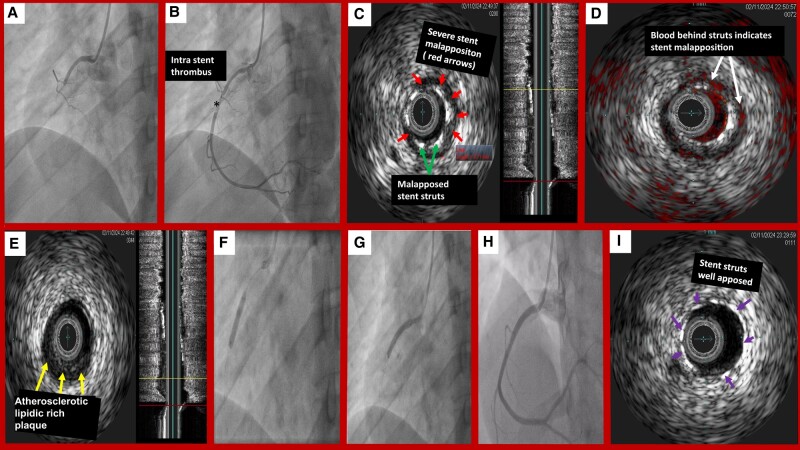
Right coronary angiography showing total acute occlusion of the mid coronary artery at the stent implantation site (stent thrombosis): white asterisk *(Panel A)* with a large intra-stent thrombus: black asterisk *(Panel B).* Intravascular ultrasound (IVUS) showing severe stent malapposition: red arrows point to locations of malapposition with a maximal malapposition length of 7 mm and clearly an undersized stent (green arrows point to stent struts) *(Panel C).* Chroma flow IVUS showing blood behind struts (white arrows) *(Panel D)*. IVUS showing Proximal lipidic rich plaque missed in the first angioplasty: yellow arrows *(Panel E).* Right coronary angiography showing overexpansion of the thrombosed stent and addition of a proximal stent *(Panel F and G)*. Achievement of a good angiographic result (*Panel H)* with good apposition of the stent struts (purple arrows point to well apposed struts) *(Panel I).*
